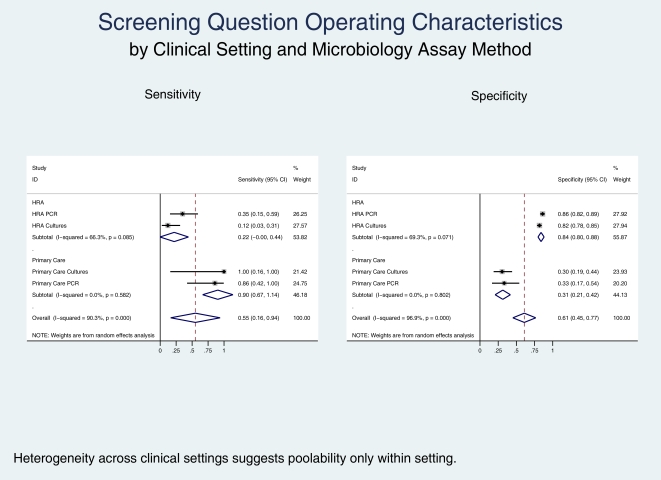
Sensitivities and specificities of screening question to predict asymptomatic anorectal STI according to clinical setting (‘primary care clinic vs. high resolution anoscopy clinic’) and microbiology assay method (‘GC culture and CT direct fluorescent antibody vs. GC/CT nucleic acid amplification test’).
